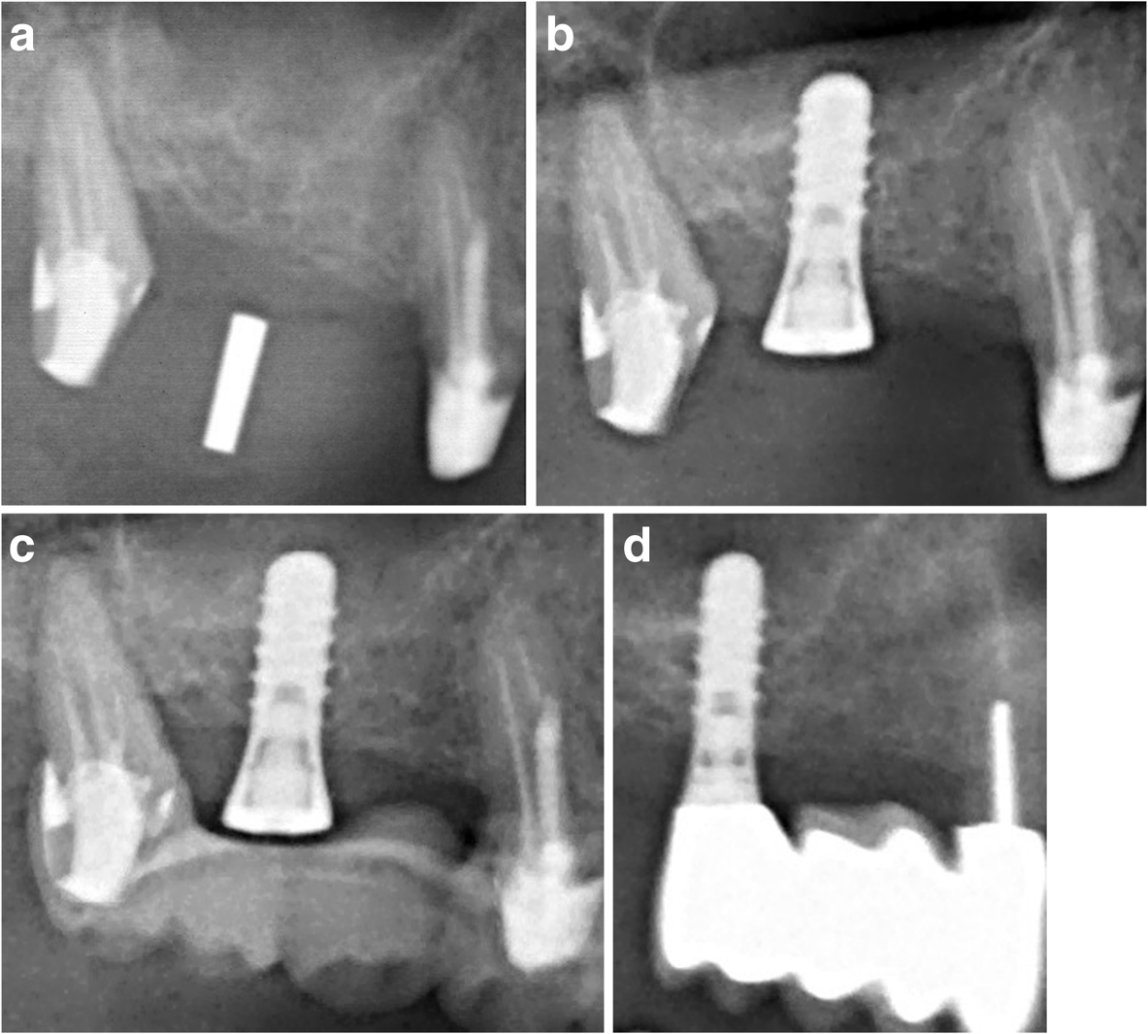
a-d Radiographs showing an exemplary participant case from the study, in a the radiologic planning is shown, b shows the radiograph after implant surgery, c shows the radiograph after 6 months and d after 10 years in clinical service
